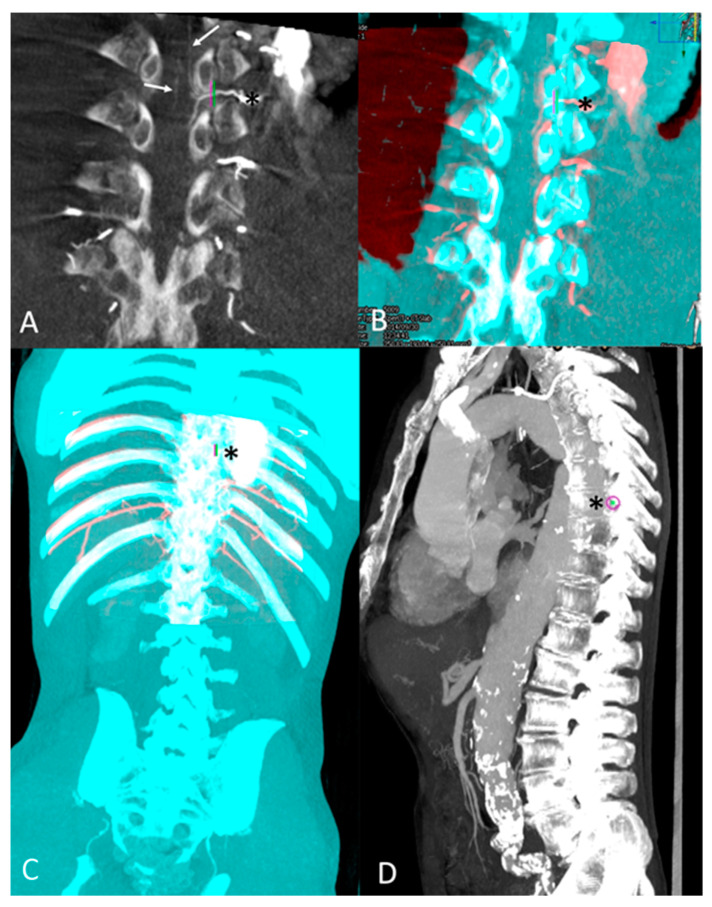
Anterior spinal detection on intra-aortic CBCT and feeder intercostal artery level tagging after 3D-3D registration with pre-operative CTA: (A) Coronal view of arterial phase CBCT with MIP reconstruction: detection of ASA (arrow). Its intercostal feeder (Asterix *) was marked by a landmark (green and purple). (B) 3D-3D semi-automatic registration with pre-procedural CTA images based on osseous structures (CBCT volume red colored; CTA volume blue colored). Its intercostal feeder (Asterix *) was marked by a landmark (green and purple). (C) Tagging of the ASA feeder, the 8th left intercostal artery (Asterix *), on an overview of overlaid CTA and CBCT volumes. (D) Global sagittal view of the entire spinal column with MIP reconstruction showing the level of 8th intercostal foramina with purple landmark (Asterix).
